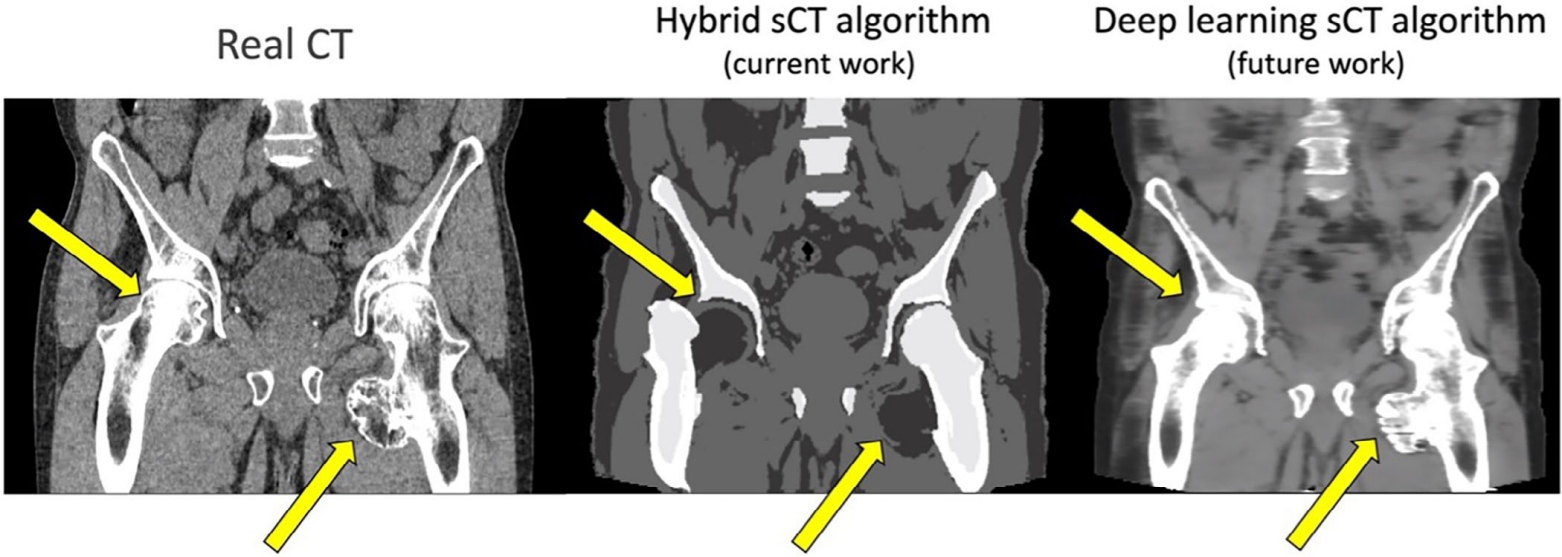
Example sagittal CT (left), sCT generated using the hybrid algorithm investigated in this study (middle), and sCT generated using the recently introduced DL‐based algorithm (right) for Patient #9 whose sCT exhibited the largest deviation in bone segmentation caused by the abnormal shape of the left femoral bone. The DL‐based algorithm appears to outperform the hybrid algorithm and will be the focus of future work. CT, computed tomography; sCT, synthetic CT.
